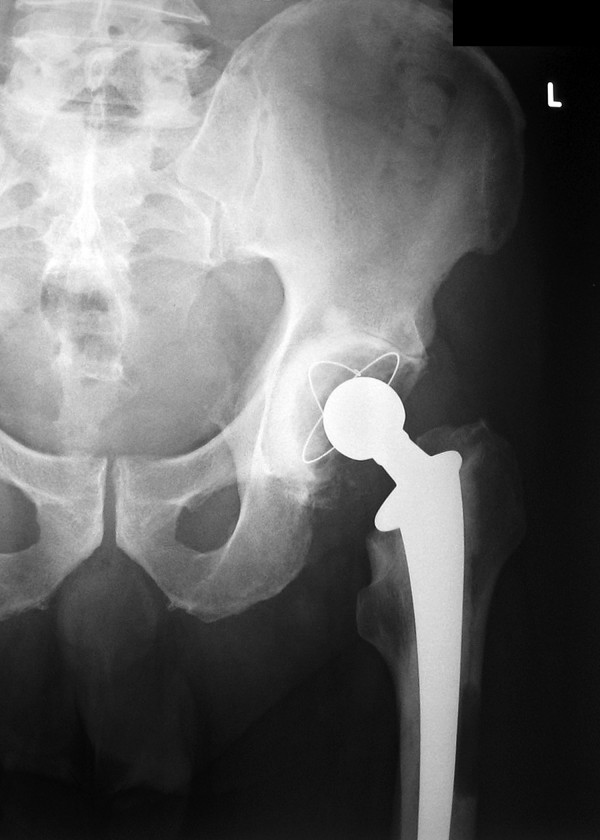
Postoperative radiograph of the left hip at 6 months showing an osteolytic lesion in Gruen zones 2 & 3 at the femoral stem.
